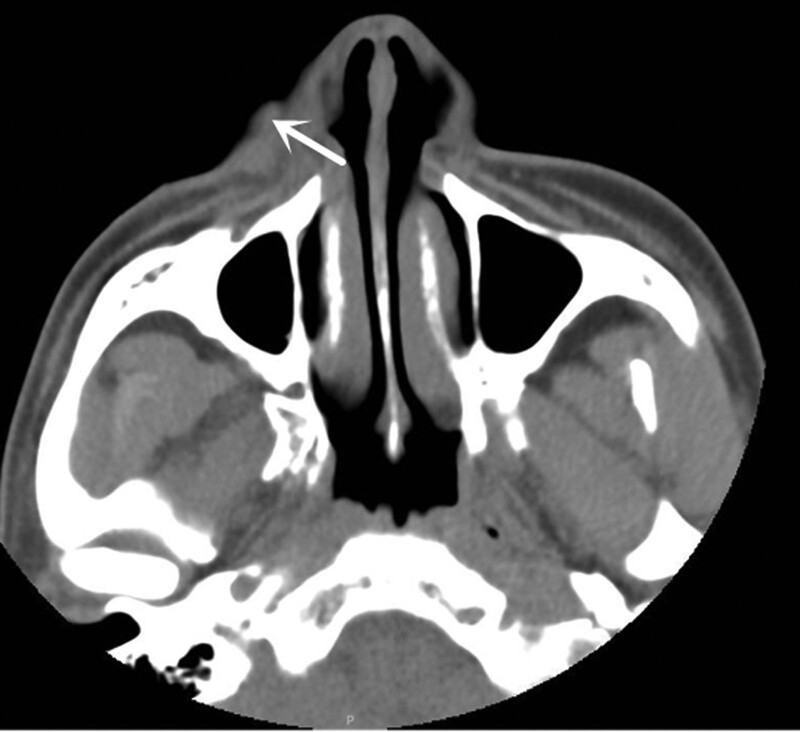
CT shows exogenous, dome-shaped nodule mass with uniform and low density with skin clear boundary (white arrow). CT = computed tomography.
